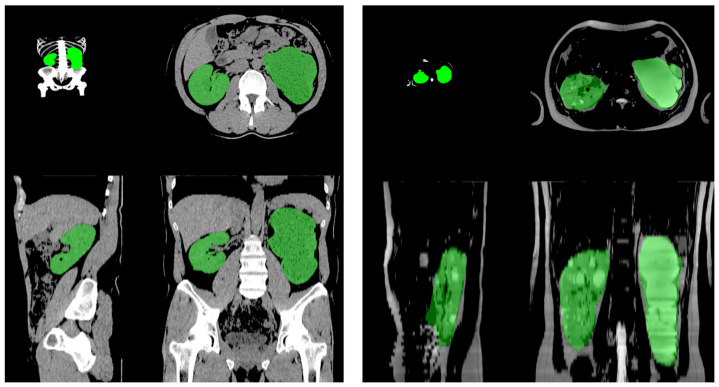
The CT scan and MRI imaging for total kidney volume calculation using AI-assisted auto-segmentation.
